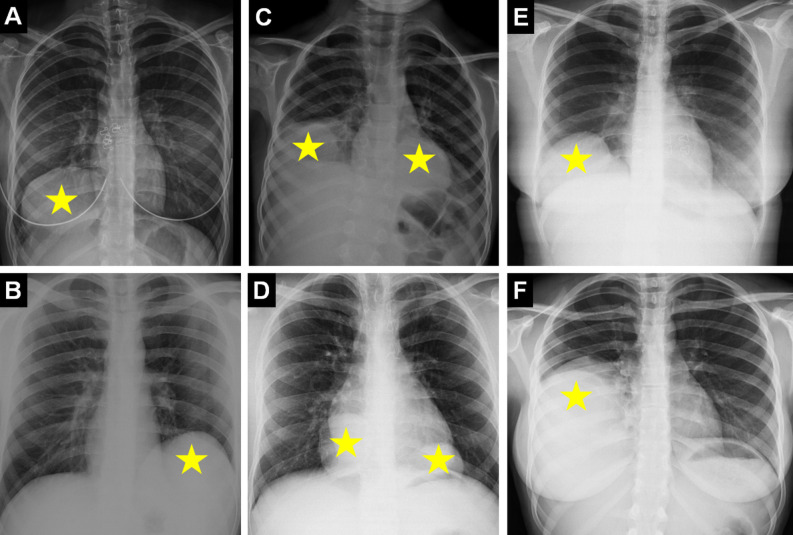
Images of cases with pulmonary hydatid cysts (HCs) located in “blind spots” (regions with high risk of being missed radiographically) on chest radiograph are indicated with asterisks. Hydatid cysts located in the right subpulmonary area (A), left subpulmonary area (B), multifocal in the right subpulmonary and left cardiophrenic angle (C), and in both cardiophrenic angles (D) are seen. The potential of breast tissue opacity to mask pulmonary HCs in female cases is seen on chest radiography (E, F).
